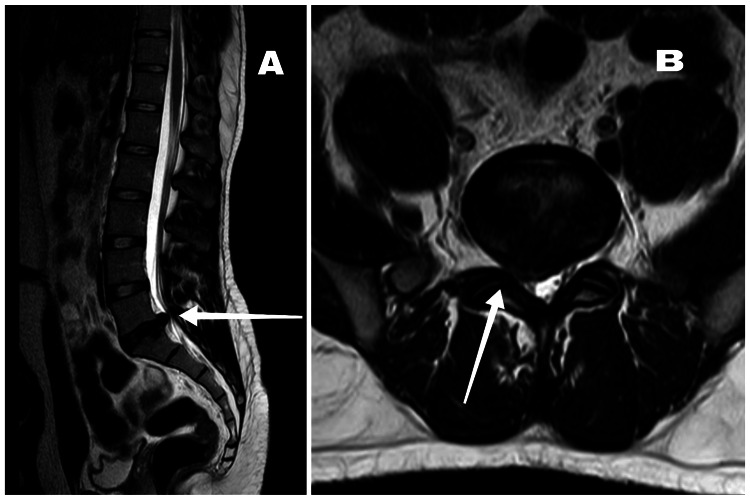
(A) Sagittal T2-weighted magnetic resonance image of lumbar spine. (B) Axial T2-weighted magnetic resonance image of spine at L5-S1 level. The arrow in image A points at the disc protrusion at L5-S1 level. The arrow in image B points at the right paracentral disc protrusion at L5-S1 level, indenting the anterior thecal sac causing significant narrowing of the spinal canal and right lateral recess.
